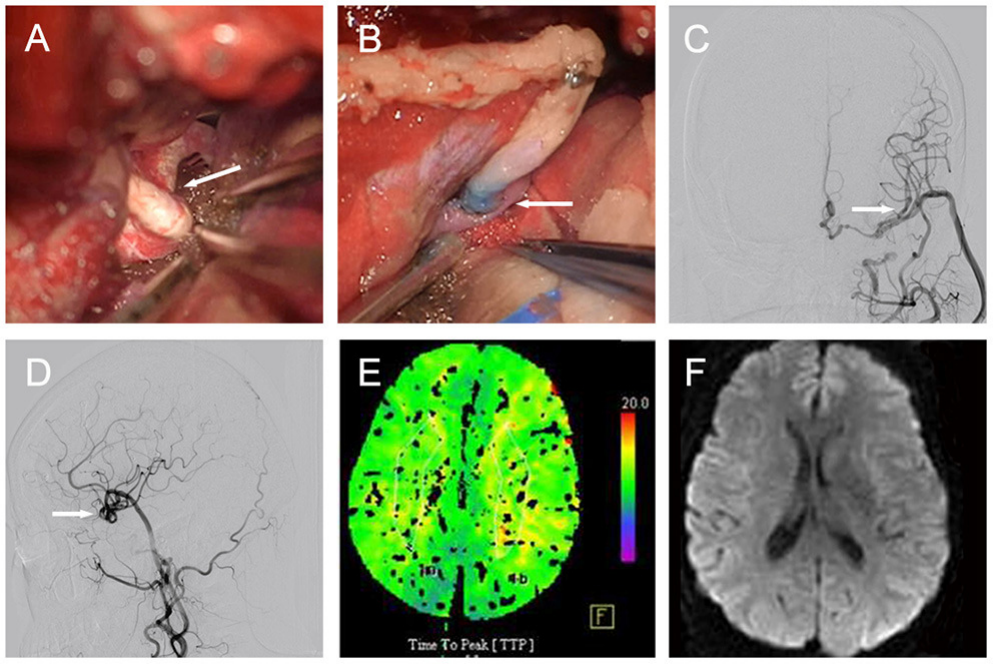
Case 1. [(A), arrow] Intraoperative image showing the left ICA constructed of double loops. [(B), arrow] PAMs treated by ligation of cervical ICA and ICA-RA-M2 bypass. Post-operative DSA demonstrating PAM disappearance and patent bypass in [(C), arrow] anteroposterior, and [(D), arrow] lateral views. (E) Axial CTP showing similar TTPs between the two hemispheres. (F) Axial DWI MRI evidencing the absence of ischemic stroke. ICA, internal carotid artery; DSA, digital subtraction angiography; PAM, pure arterial malformation; RA, radial artery; DWI, diffusion weighted imaging; MRI, magnetic resonance imaging; TTP, time to peak; CTP, computed tomography perfusion.
